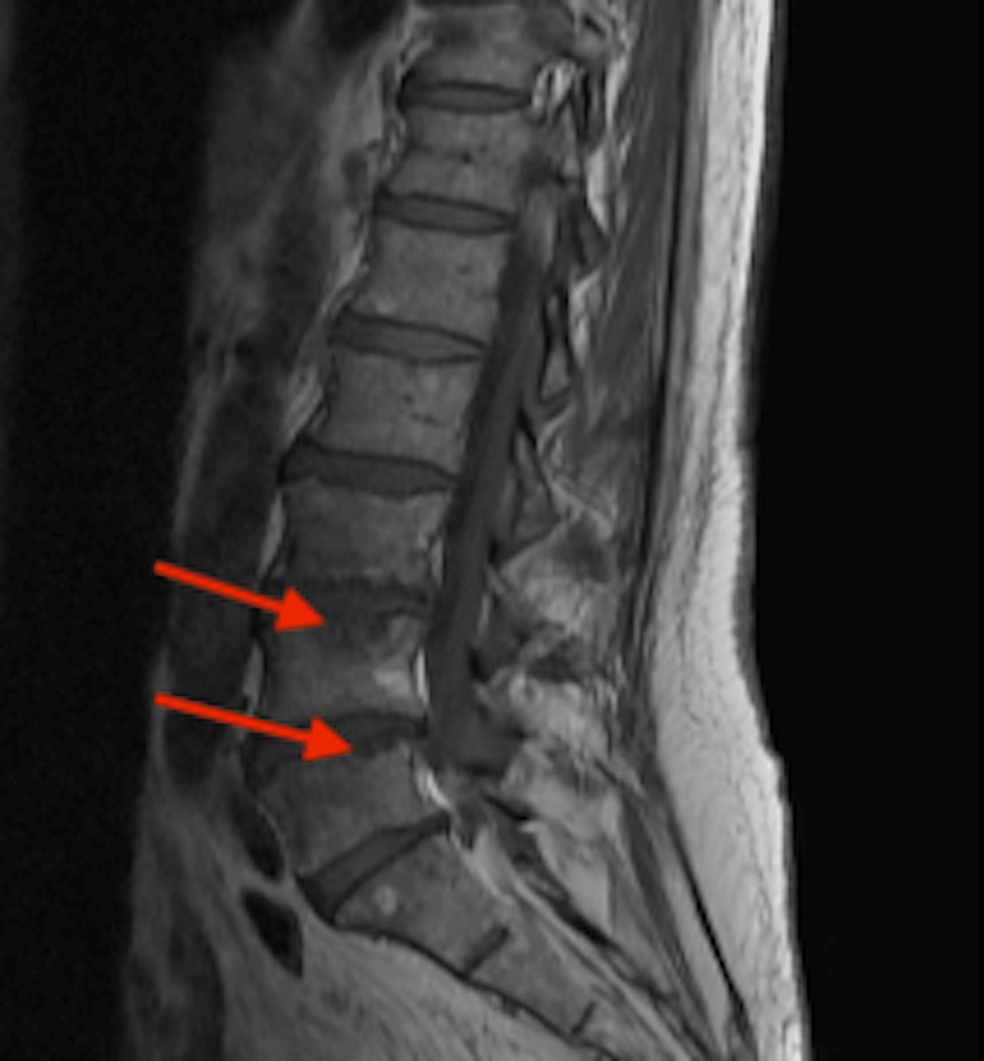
Magnetic resonance imaging depicts discitis L4-5 vertebrae (red arrows).
